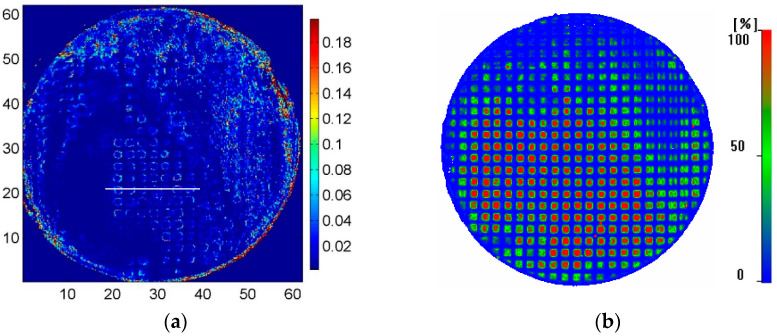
Nonlinear and linear ultrasonic C-scan images: (a) nonlinear ultrasonic C-scan; (b) linear ultrasonic C-scan.
